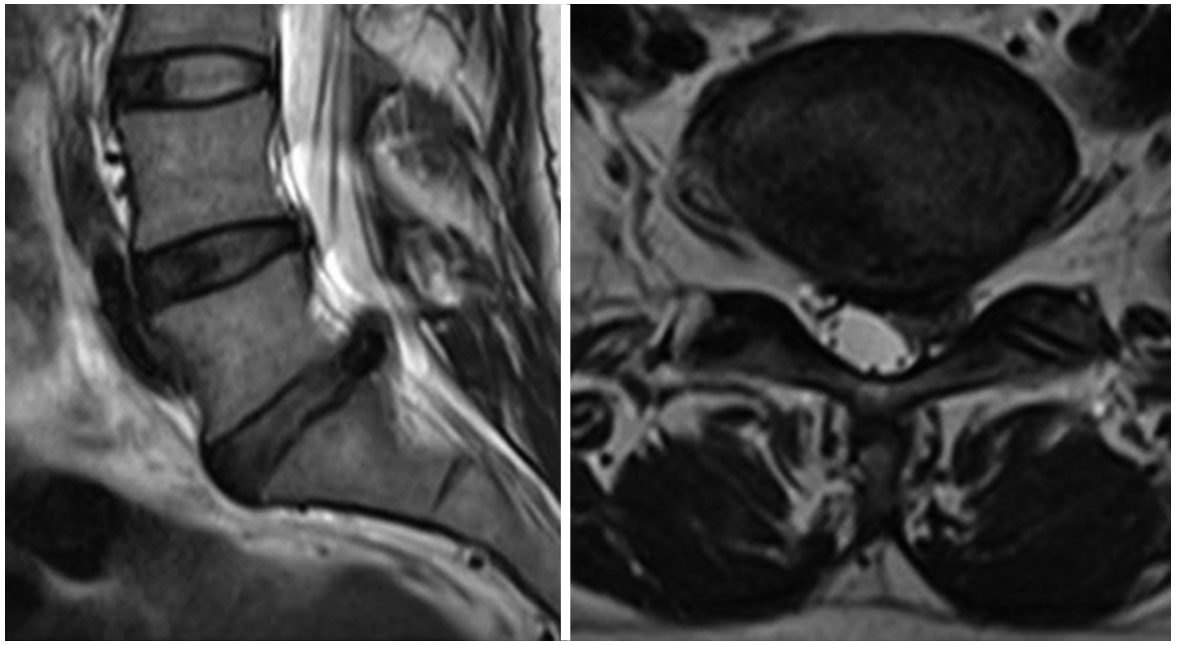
Preoperative MRI showing a left paramedian extruded disc herniation at L5-S1 compressing the dural sac and left S1 nerve root in the lateral recess (T2-weighted sagittal and axial views)
